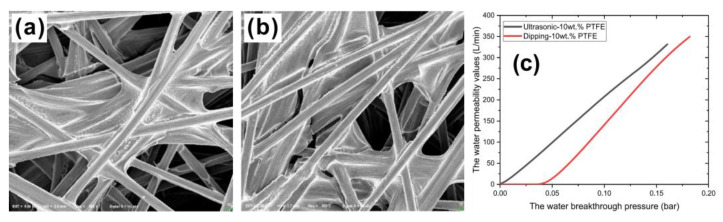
SEM images of the samples prepared with (a) the ultrasonic dispersion method, and (b) the direct dipping method. (c) Performance curves plotted with the water permeability values against water breakthrough pressure. Reproduced with permission [78]. Copyright 2022, Elsevier.
